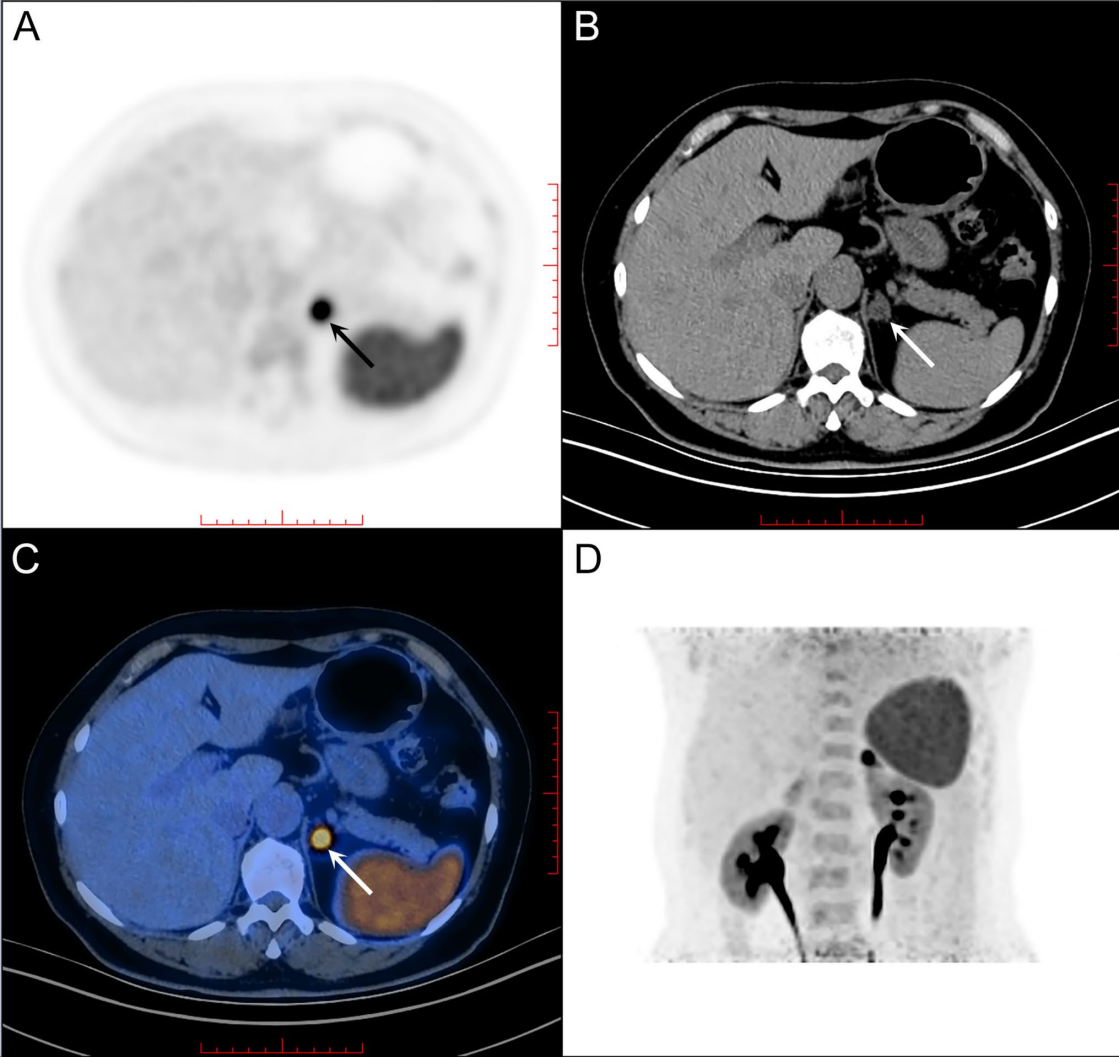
The performance of ^68^Ga-Pentixafor PET/CT imaging in PA patients. A 60-year-old woman presented with hypertension and hypokalemia of 2.45mmol/L. CT scan showed a nodule of 1.9 cm and the second nodule of 0.9 cm in the left adrenal gland, and another adrenal lesion of 1.0 cm on the right side. AVS indicated left aldosterone hypersecretion, with the lateralization index of 13.1 (> 2). (B) CT scan showed a nodule of 1.9 cm. (A, C, D) Positive findings of ^68^Ga-Pentixafor PET/CT demonstrated strong uptake of ^68^Ga-Pentixafor on the left adrenal lesion, with SUV_max_ of 13.9 in the nodule of 1.9 cm, which was in accordance with the findings of AVS. Left adrenalectomy was performed, and the plasma potassium increased to 4.64 mmol/L without oral supplement. PA, primary aldosteronism. AVS, adrenal vein sampling. (CT scan and 68Ga-Pentixafor PET/CT of the 0.9 cm nodule in the left adrenal gland and the 1.0 cm nodule on the right side are shown in Supplementary Material as Supplementary Fig. 2 & Fig. 3)
